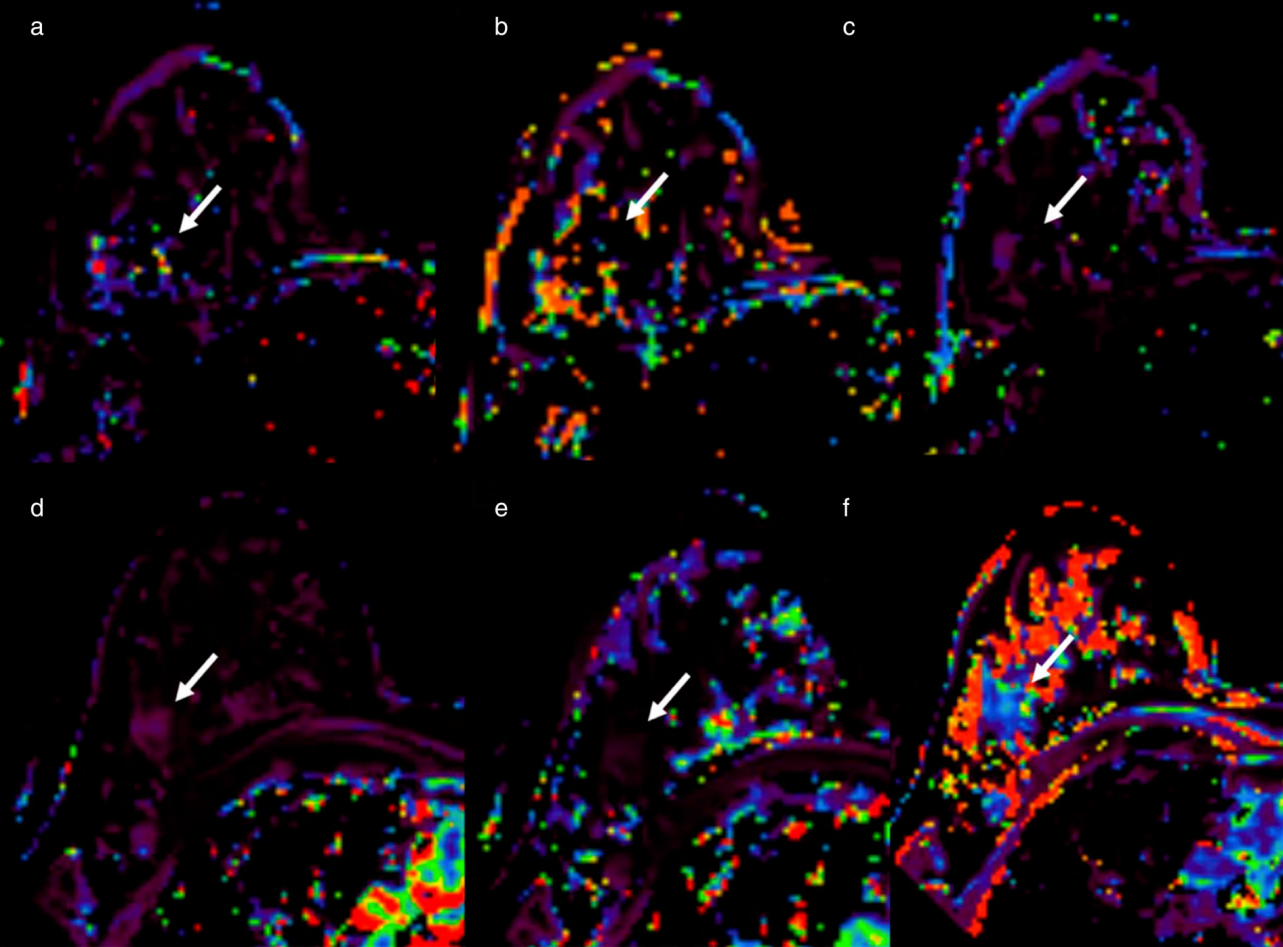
Example of early assessment of the response to NAC using dynamic contrast‐enhanced imaging (DCE‐MRI). 37‐year‐old patient with a G3, triple negative invasive ductal carcinoma of the right breast (arrows, same case shown in Figure 4). (a–c) = pre‐NAC examinations; (d–f) = early assessment examination after two cycles of cytotoxic NAC. Ktrans (a and d), Kep (b and e), and Ve (c and f) maps. Early assessment showed a reduction of Ktrans (286 vs. 83.9 min^−1^) and kep (91.49 vs. 20.14 min^−1^ × 100) with a slight increase of Ve (275.34 vs. 308.08 × 1000) signal intensity on ADC maps (c) compared to the pre‐treatment examination (f). Pathological complete response was proved at pathology examination after surgical resection. Reprinted under a Creative Commons (CC BY 4.0) license from: Romeo V, Accardo G, Perillo T, Basso L, Garbino N, Nicolai E, Maurea S, Salvatore M. Assessment and Prediction of Response to Neoadjuvant Chemotherapy in Breast Cancer: A Comparison of Imaging Modalities and Future Perspectives. Cancers (Basel). 2021;13(14):3521.
